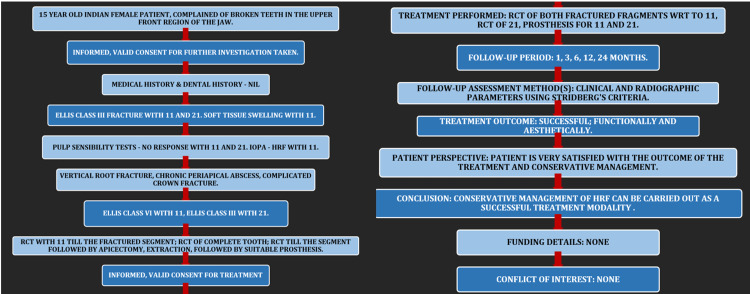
PRICE 2020 guidelines depicting the steps involved in reporting this case report. IOPA: intra-oral periapical radiograph, HRF: horizontal root fracture, RCT: root canal treatment.
